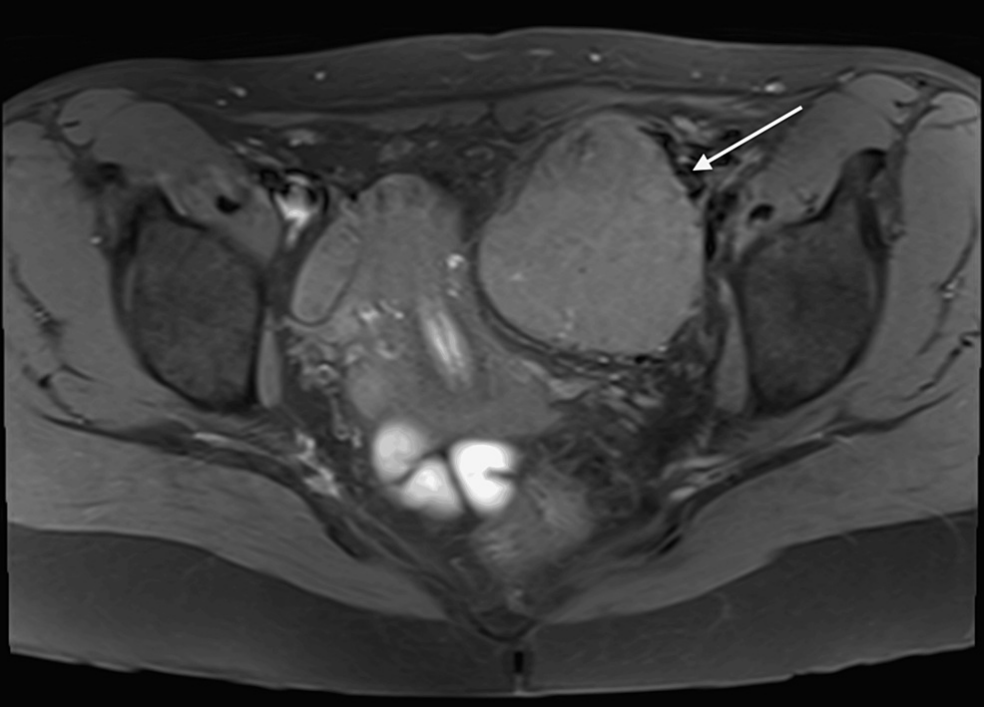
MRI T1 axial pelvis showing the left pelvic sidewall (IDCS) (white arrow) MRI: Magnetic resonance imaging; IDCS: Interdigitating dendritic cell sarcoma
